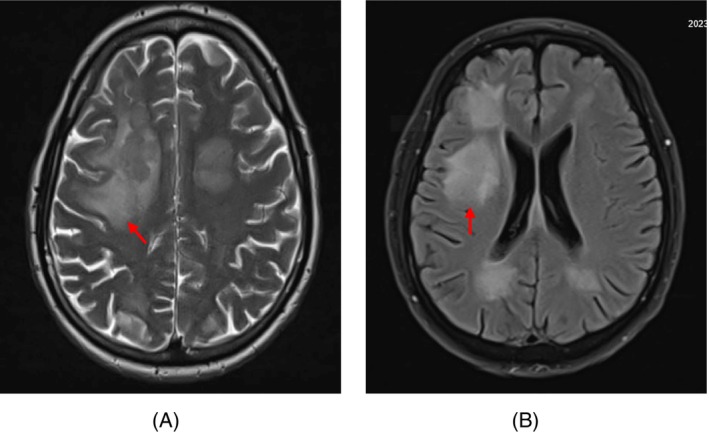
Multiple vasogenic regions of edema as high T2/FLAIR signal in both hemispheres of the brain. T2 sequence (A), T2/FLAIR sequence (B), red arrow.
